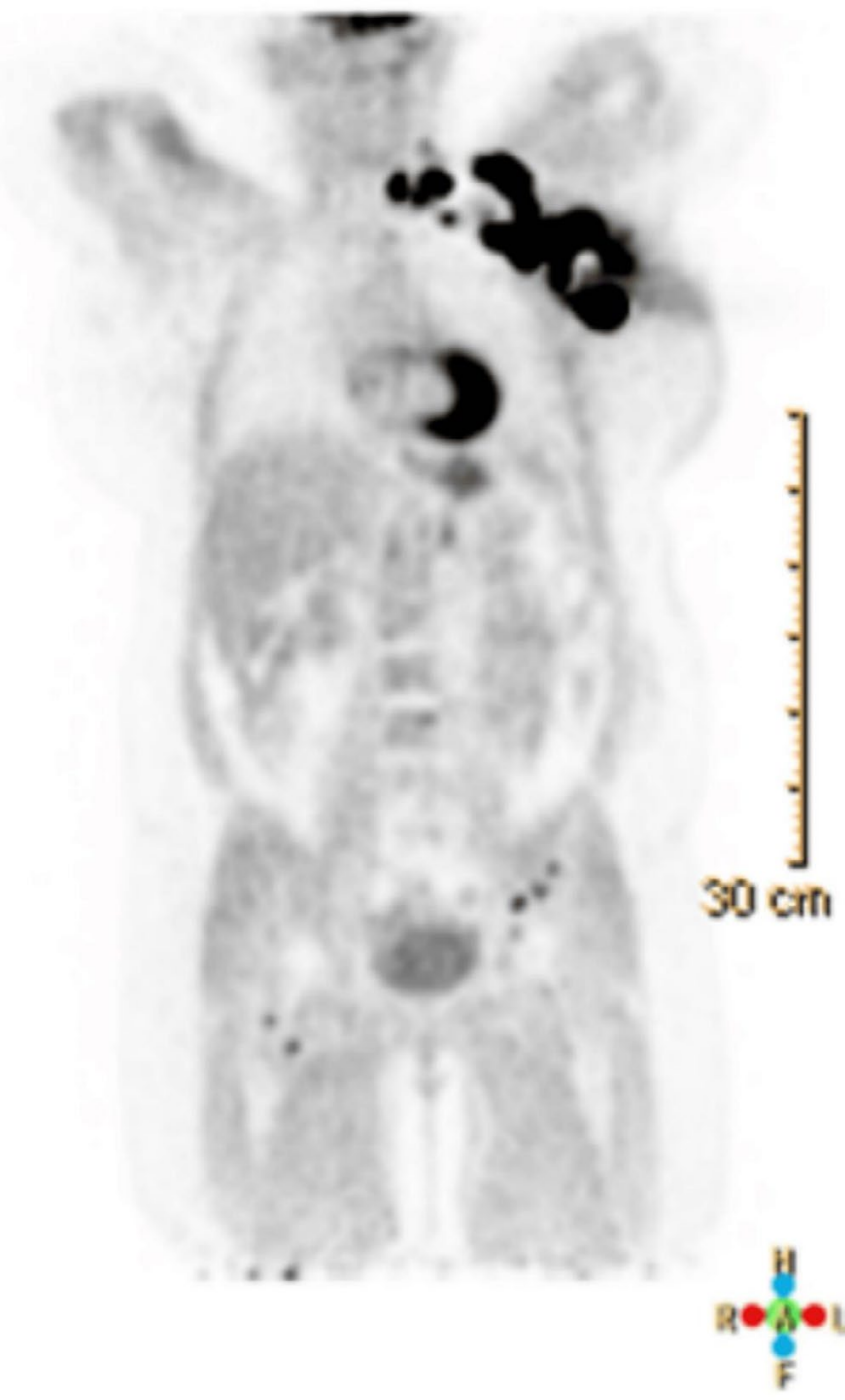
PET/CT scan revealing extensive metastatic disease. PET/CT taken two months after mammogram and ultrasound demonstrated a 4.6 × 4.0 × 5.2 cm hypermetabolic, irregular mass in the left breast, corresponding to the patient's biopsy‐proven invasive ductal carcinoma. Multiple enlarged, irregular, and necrotic‐appearing metastatic lymph nodes were visualized, largest at the left axilla, subpectoral, and infraclavicular regions. Scattered osseous metastases were noted throughout the axial skeleton and proximal bilateral femurs.
